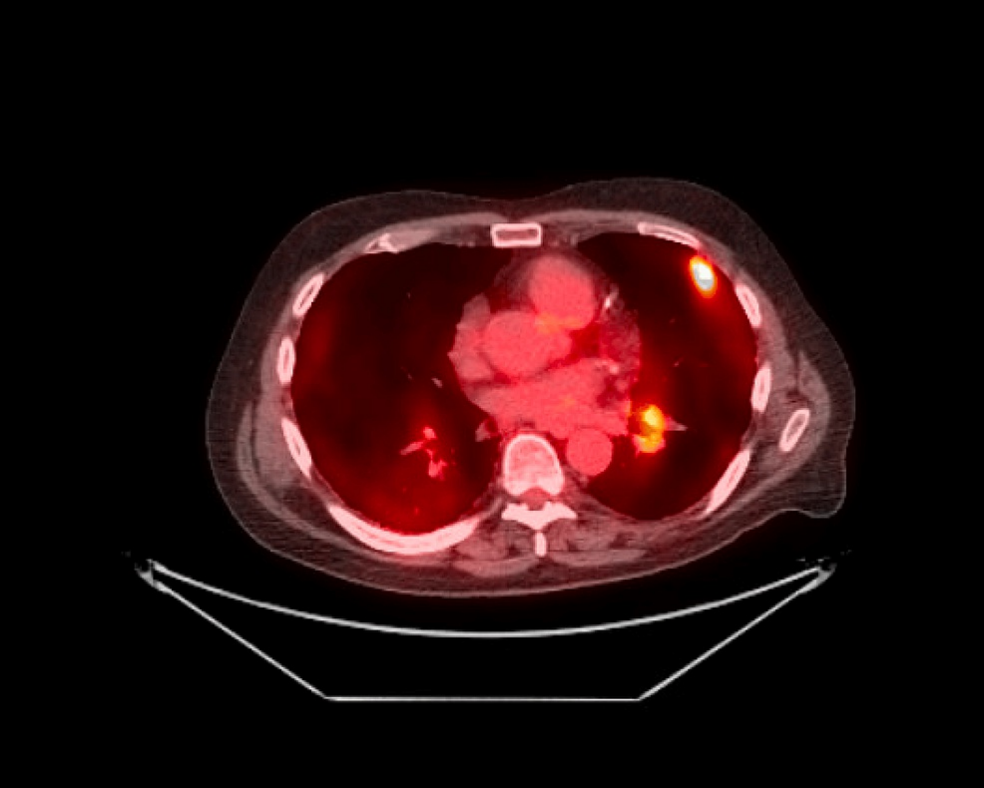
Initial PET-CT scan The positron emission tomography-computed tomography (PET-CT) scan image is showing bright areas corresponding to hyper-metabolic activity in left hilar lymph node and left lower lung base which appeared to be the primary location of lung carcinoma.
